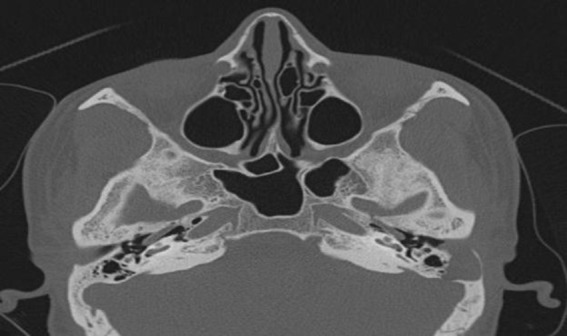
HRCT Temporal Bone revealing erosion of posterior wall of external auditory canal by Cholesteatoma
